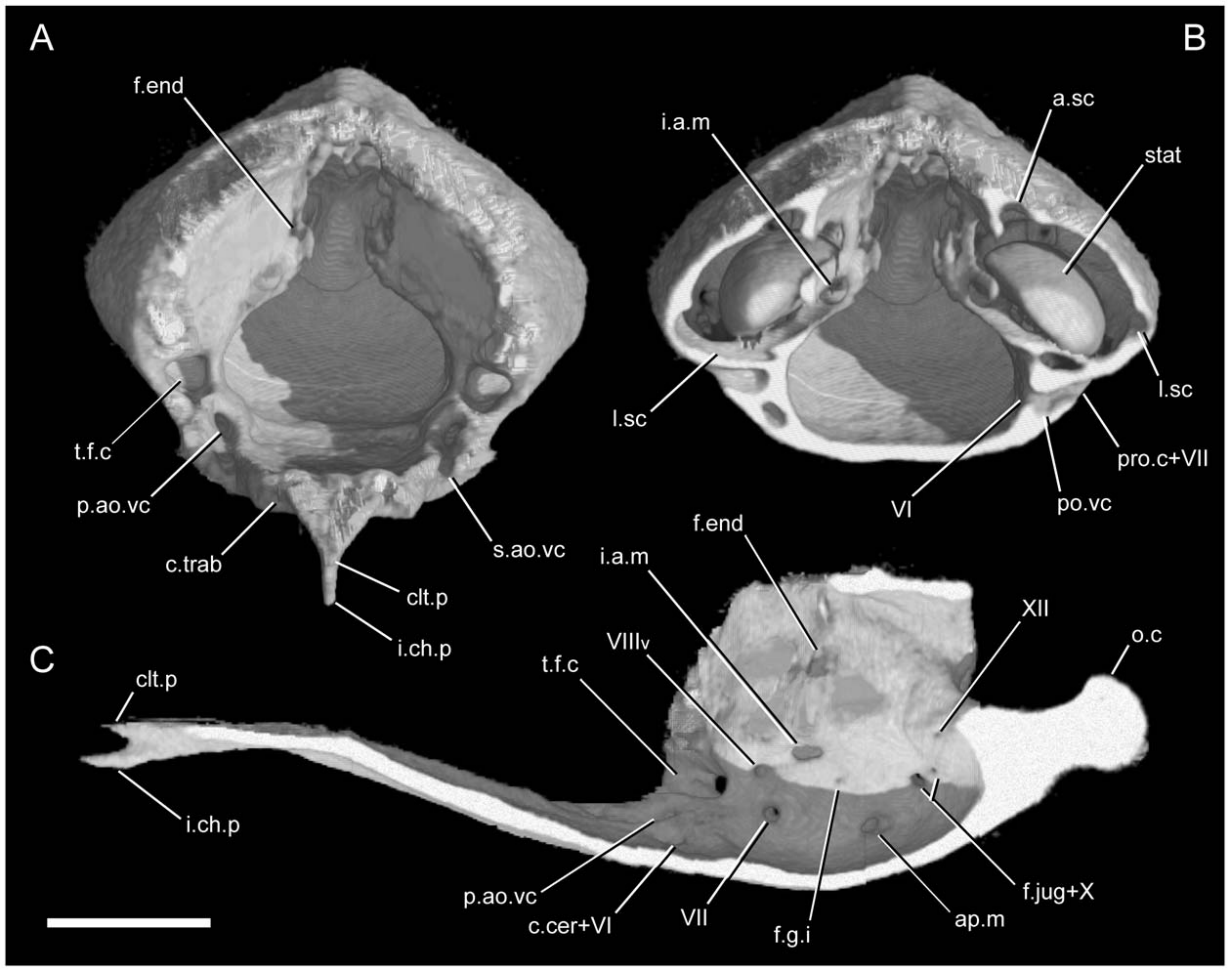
CT images of the otooccipital complex of *Uropeltis woodmasoni* (TMM M-10006). Scale bar = 1.0 mm. (A) Anterior view; (B) Anterior cut-away at level of otic capsule; (C) Lateral view, anterior to left. a.sc = anterior semi-circular canal; ap.m = apertura medialis recessus scalae tympani; c.cer = cerebral carotid foramen; c.trab = ossified crista trabecularis; clt.p = cultriform process; f.end = endolymphatic foramen; f.jug = jugular foramen; i.a.m = internal auditory meatus; i.ch.p = interchoanal process of sphenoid; l.sc = lateral semi-circular canal; o.c = occipital condyle; p.ao.vc = primary anterior opening of vidian canal; po.vc = posterior opening of vidian canal; pro.c = prootic canal; s.ao.vc = secondary anterior opening of vidian canal; s.cir = semi-circular canal; stat = statolithic mass; t.f.c = trigeminal facialis chamber (for trigeminal branches two and three); VI = abducens nerve; VII = facial nerve seven (to prootic opening); VIIIv = vestibular branch of auditory nerve; X = vagus nerve; XII = hypoglossal nerve.
